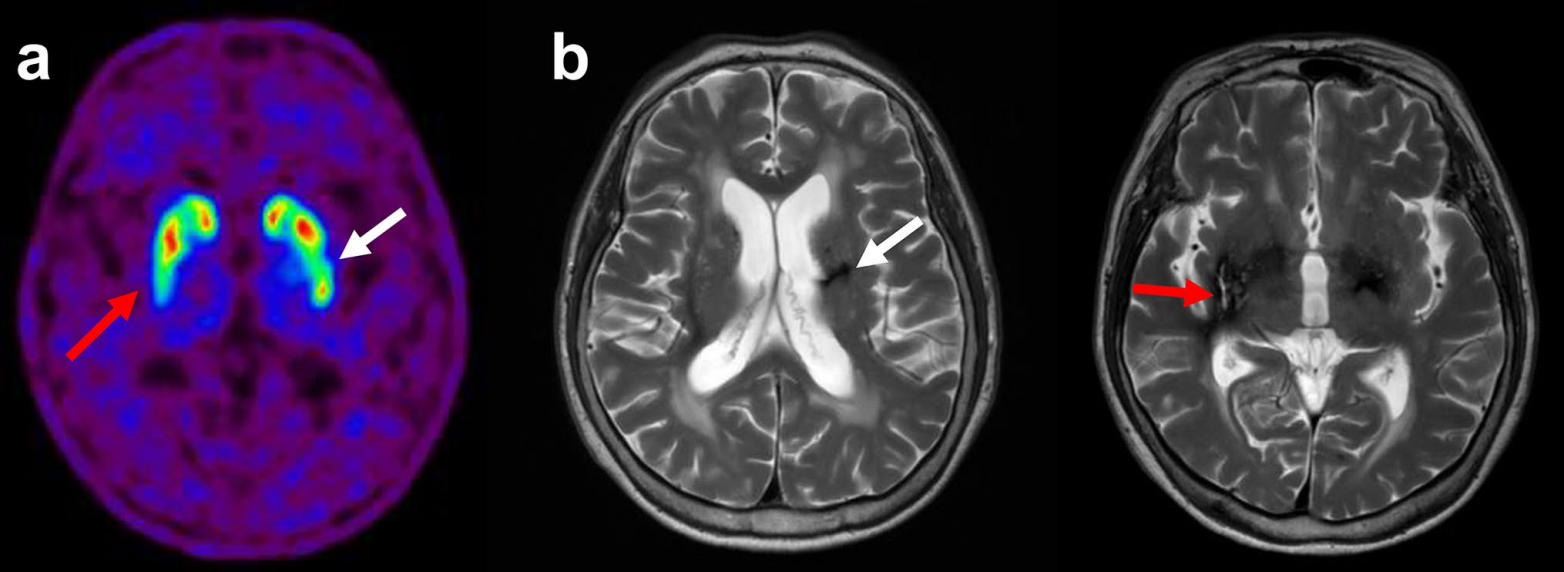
Representative images of vascular parkinsonism with ischemic lesions-related changes. Transaxial [^18^F]FP-CIT PET (a) shows focally reduced uptake in bilateral putamens with corresponding an old striatocapsular infarction (white arrows) and an old hemorrhagic infarction (red arrows) on T2 weighted MR images (b)
